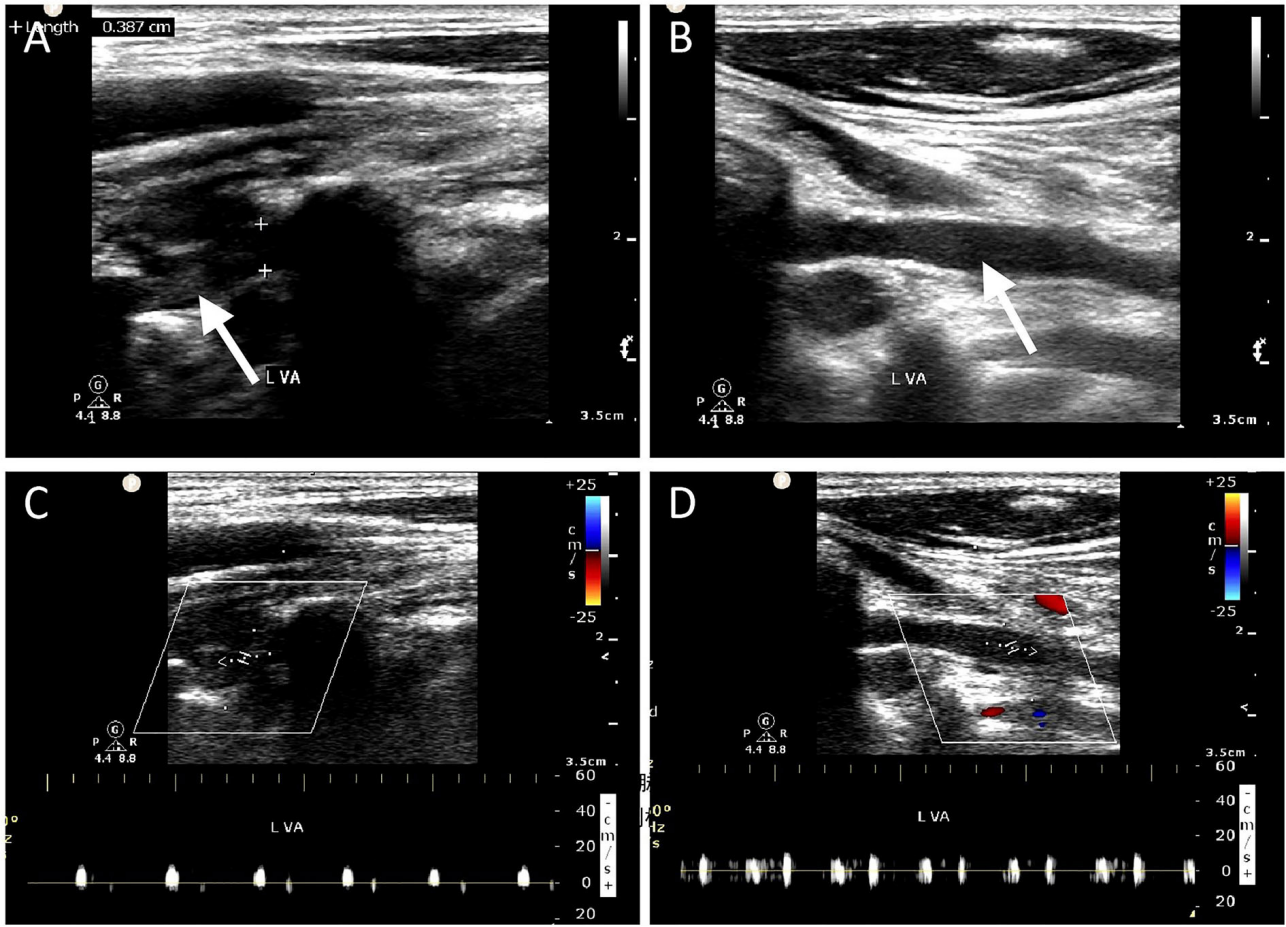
Ultrasonographic imaging for one case (Patient # 5) with left vertebral artery dissection. The 40-year-old man who had a history of falling down from a freight car 1 day ago presented with sudden syncope on admission. (A) Gray scale ultrasound examination reveals heterogeneous echo in the lumen, indicating intraluminal thrombus formation, which occupies the entire lumen of the V2 segment. (B) A homogenous echo in the V1 segment is suggestive of intramural hematoma. (C,D) No blood flow signal was detected, suggesting occlusion of the left VA.
